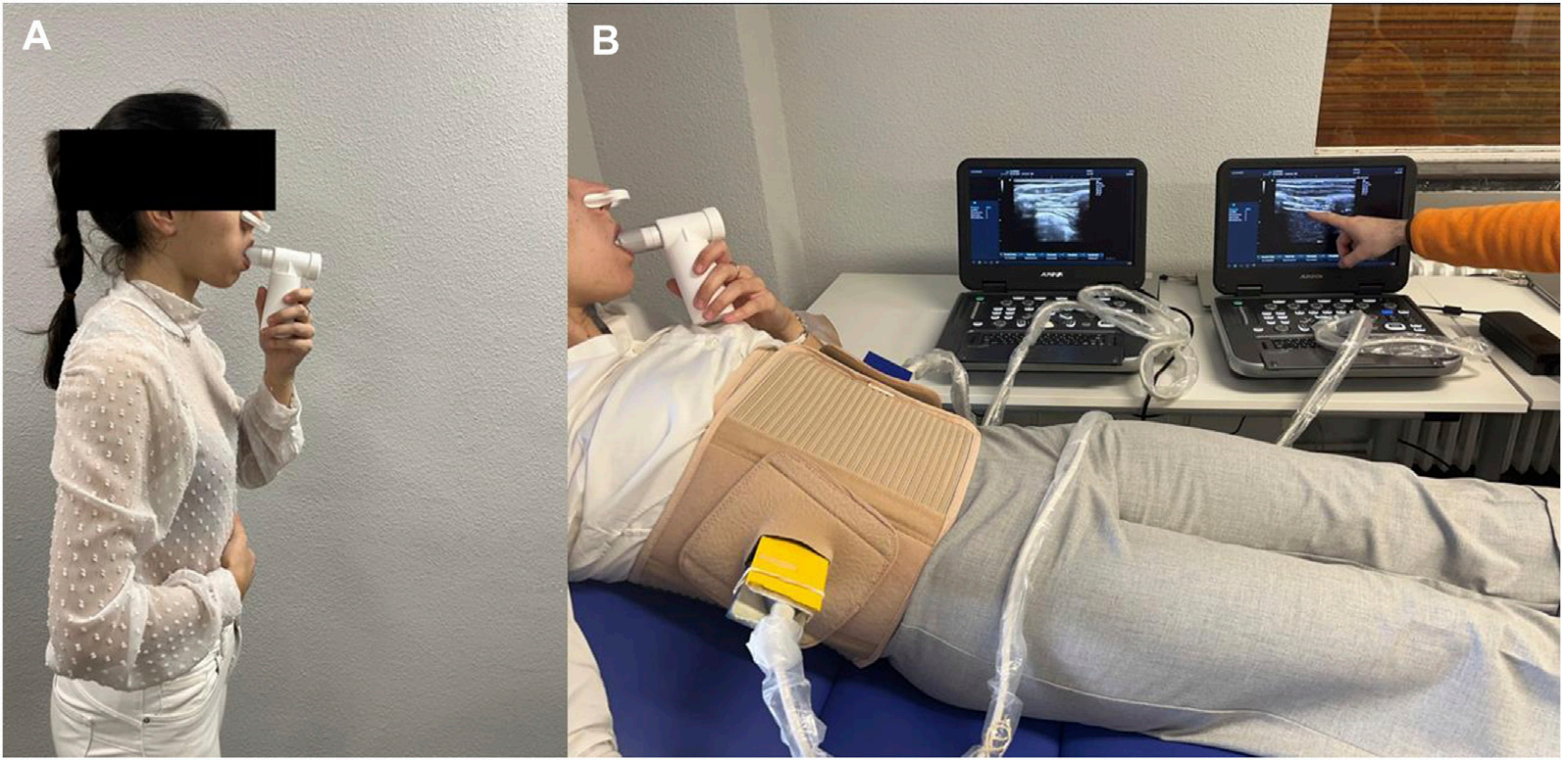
(A) High-intensity inspiratory muscle self-training (IMT) using the POWERbreathe device from Medic. (B) Simultaneous bilateral ultrasonography visual biofeedback within the proposed orthosis device using two holding devices to fix the right and left ultrasound probes perpendicular to the last intercostal space following the right and left mid-axillary lines.
